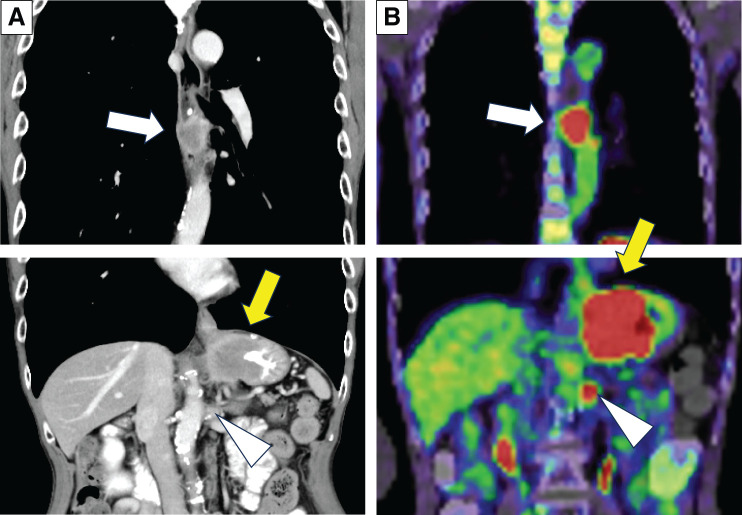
Images of CT, and PET-CT. CT showing an approximately 3- cm primary tumor in the middle esophagus (white arrows), along with enlarged para-aortic lymph node (arrow heads) and lymph nodes in the lesser curvature of the stomach (yellow arrows), which were suspected to have invaded the stomach wall (A). PET-CT showing increased uptake in these areas, suggesting metastases from esophageal cancer (B).
